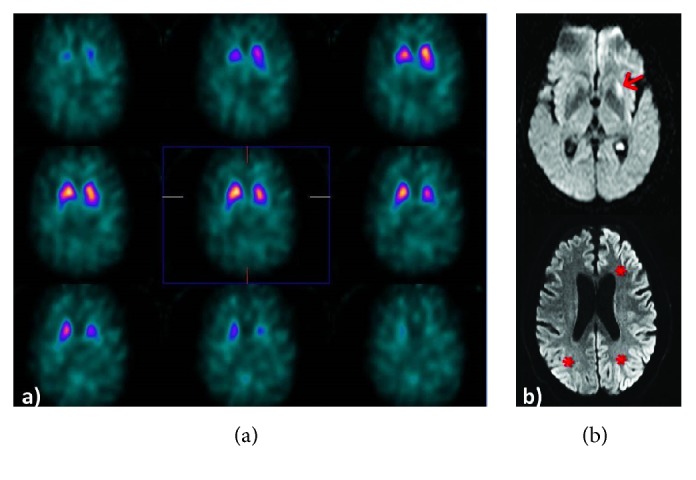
(a) DaTSCAN detected a slight decrease in tracer uptake in the left caudate nucleus, as well as reduced uptake in the putamina (more marked in the left). (b) Brain MRI scan (axial, diffusion-weighted sequences). Above: hyperintensity in left putamen (arrow). Below: disease progression was associated with involvement of the cortex of the left hemisphere and, to a lesser extent, the right temporoparietal cortex (asterisks). Images were taken at a one-month interval.
